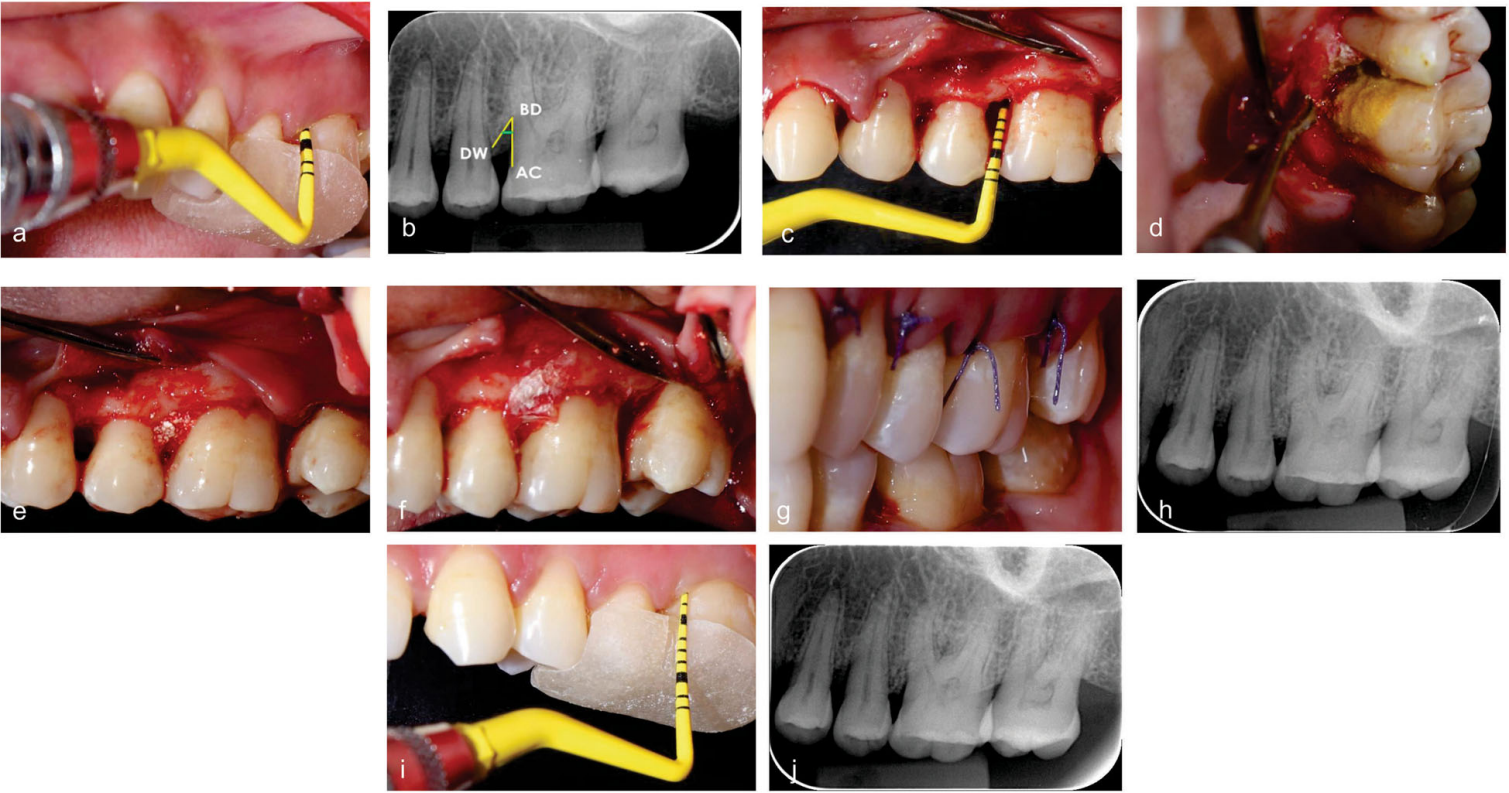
a Pre-operative clinical images with acrylic stent in place to measure the PPD, CAL in the region of mesiobuccal [8(9) mm]. b Preoperative digital intra oral radiograph with intrabony defect in relation to mesial aspect of 26. c Simplified papilla preservation done in relation to mesial of 26 and flaps raised, Intra-operative clinical measurement of the two wall defect from the CEJ to the base of the defect in relation to mesiobuccal aspect of 26 = 5mm. d Root conditioning done with tetracycline in relation to 26. e Defect site grafted with B-ostIN HT (HA60 %:BTCP40 %). f Amniotic membrane placed over the grafted site in relation to mesial aspect of 26. g External vertical mattress suturing done with 4 − 0 vicryl sutures. h Immediate post-operative digital intra oral radiograph showing complete defect fill in relation to mesial aspect of 26. i Six months post-operative clinical images with acrylic stent in place to measure the PPD, CAL in the region of mesiobuccal [2(3) mm]. j Six months post-operative RVG in relation to mesial aspect of 26 showing complete defect fill
